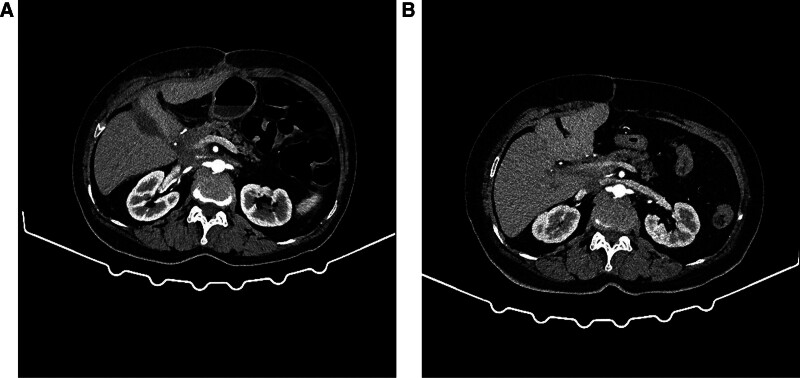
Follow-up abdominal contrast-enhanced CT scans. Both scans demonstrate no evidence of significant abnormalities. (A) One-month postoperative scan. (B) Scan from January 3, 2024. CT = computed tomography.
